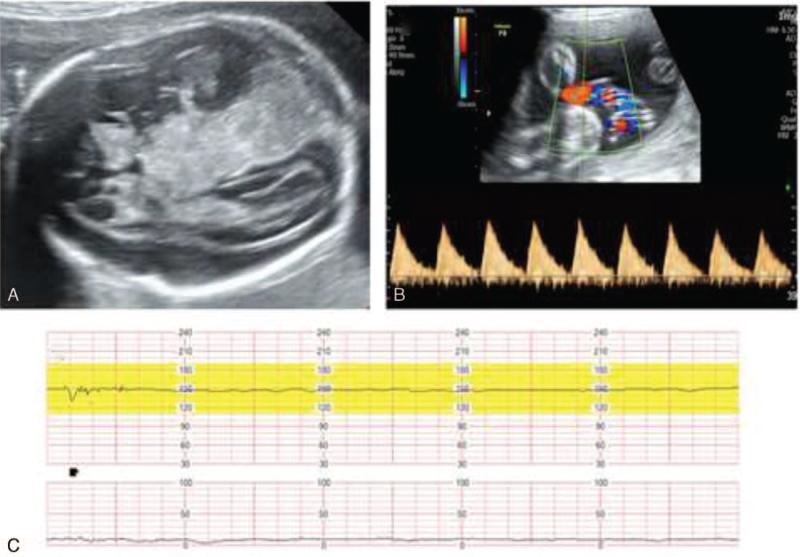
Six hours after diagnosis of fetal brain hemorrhage. (A) Ultrasonography of fetal brain. Massive fetal intracranial hemorrhage was observed. (B) Doppler ultrasonography of umbilical artery, indicating absent diastolic flow. (C) Nonstress test (NST) showing minimal fetal variability.
